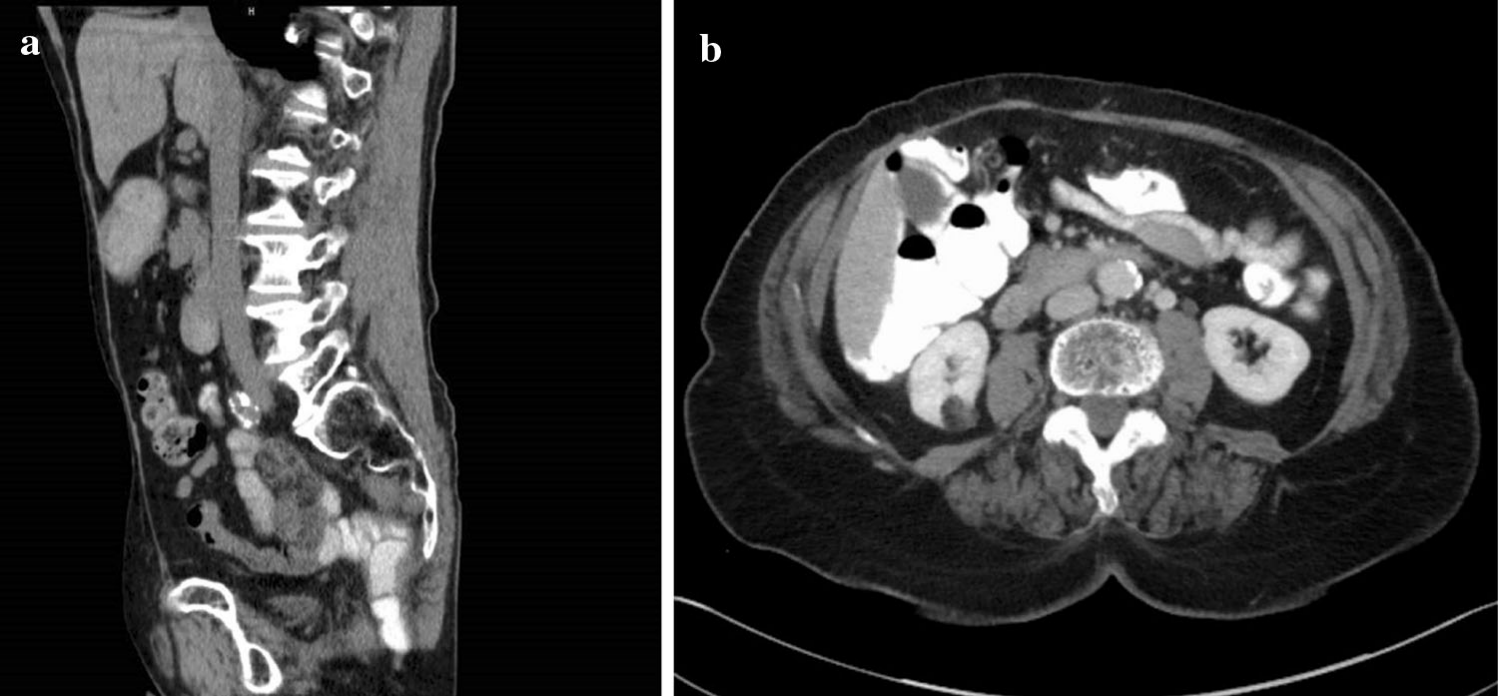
Sagittal (a) and axial (b) computed tomography scans of a stable abdominal wall 33 months after donor site closure with a doubled Vypro® mesh
